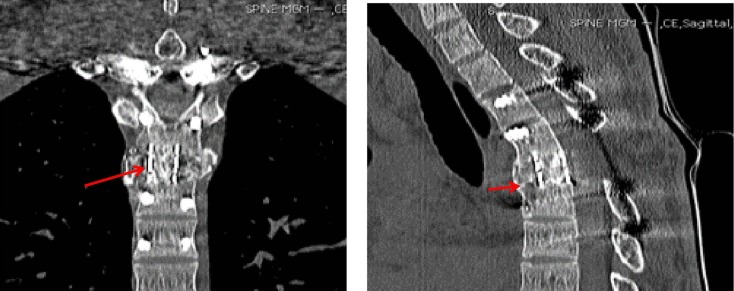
Postoperative MRI showing new bone formation at 18 months Magnetic resonance imaging (MRI).
